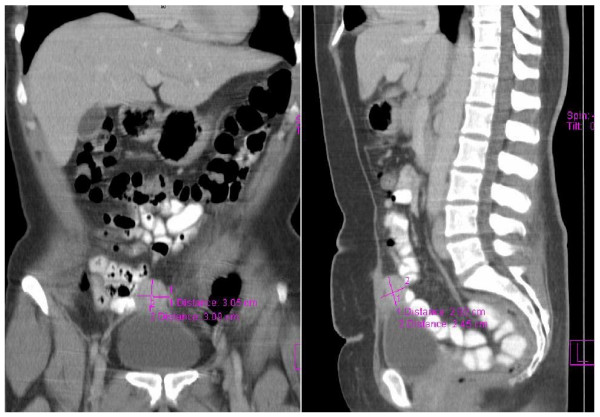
Selected computed tomography images showing urachal endometrioma.
